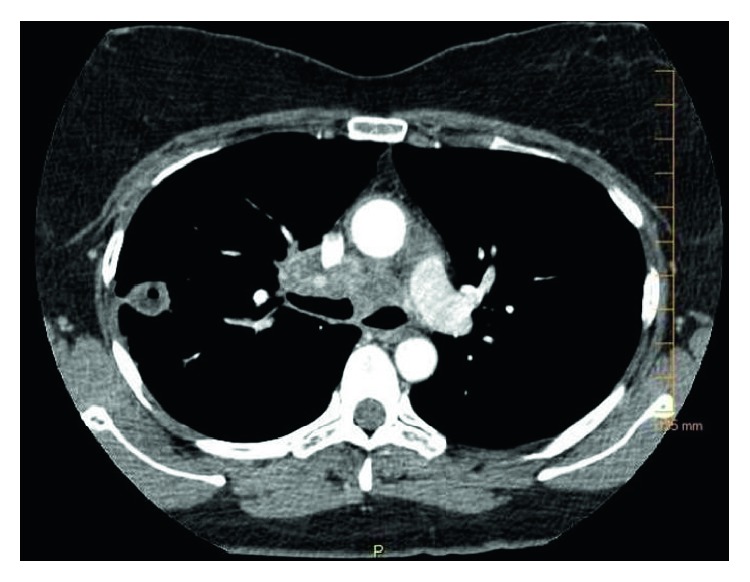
CT chest showing large necrotic mass.
